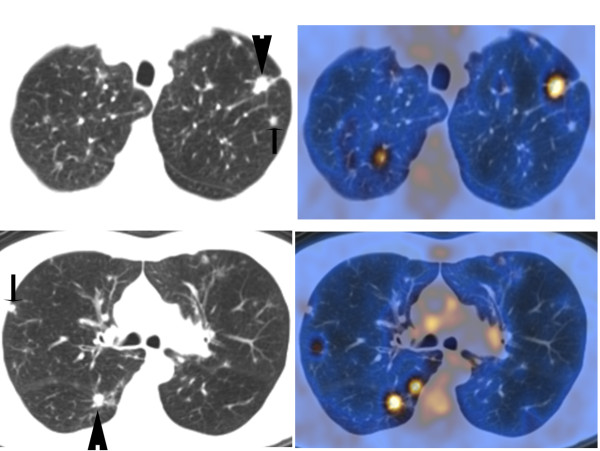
PET findings in nodular PLCH. The chest CT images on the left upper and lower panels show multiple lung nodules in a smoker with surgical lung biopsy-proven PLCH. The corresponding PET images on the right upper and lower panels show PET characteristics of the multiple pulmonary nodules. The larger pulmonary nodules (arrowhead) demonstrated intense PET uptake, while other nodules (arrow) are PET-negative (Standardized Uptake Value < 2.5).
